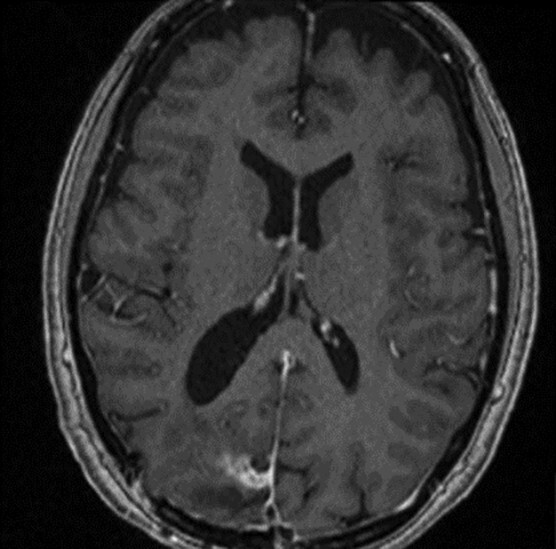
T1 weighted axial MRI post-gadolinium enhancement 8 weeks postdischarge. Right occipital postsurgical appearances are again demonstrated with reduction in the extent of vasogenic oedema. The enhancing rim that was present previously has retracted, with only focal curvilinear enhancement now evident in the right occipital lobe. These appearances are likely postsurgical/treatment related, and there is no convincing residual or recurrent disease.
